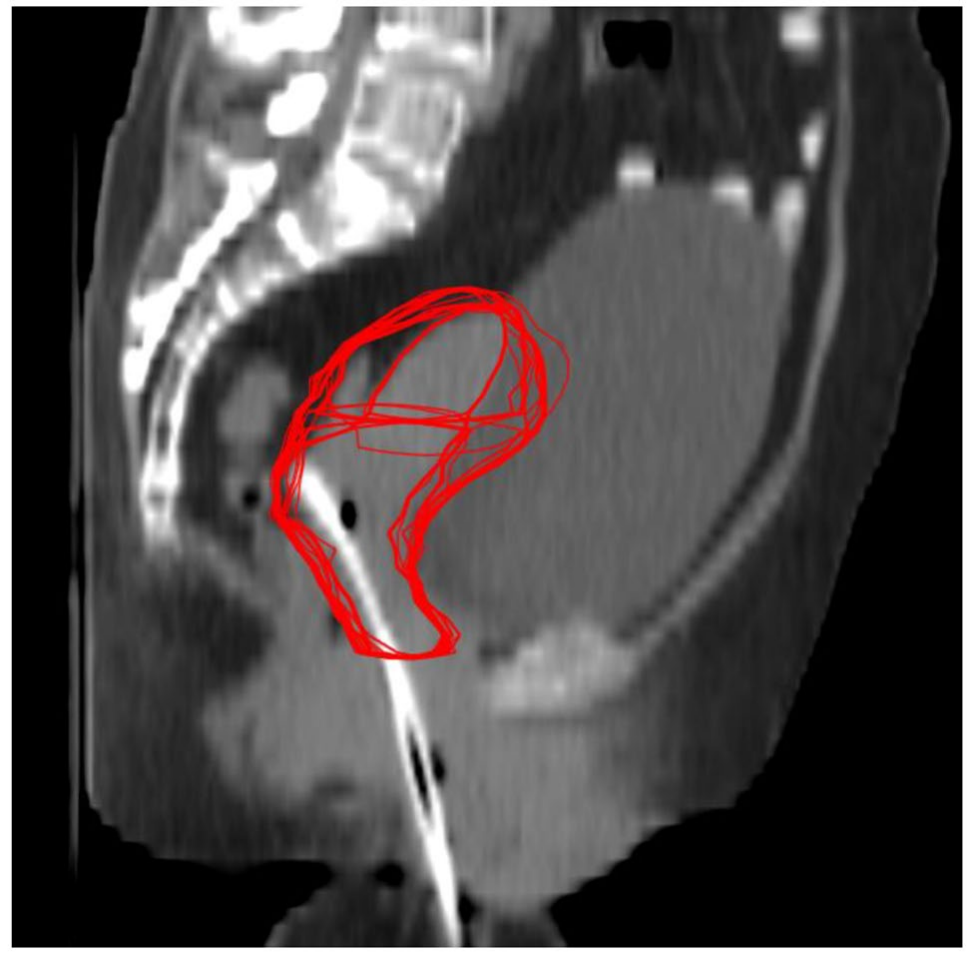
Sagittal image taken from a planning CT scan of one patient. All the CTV-Us and CTV-Cs (red) contours from the iCBCT were mapped to the planning CT after rigid registration with respect to bony anatomy
